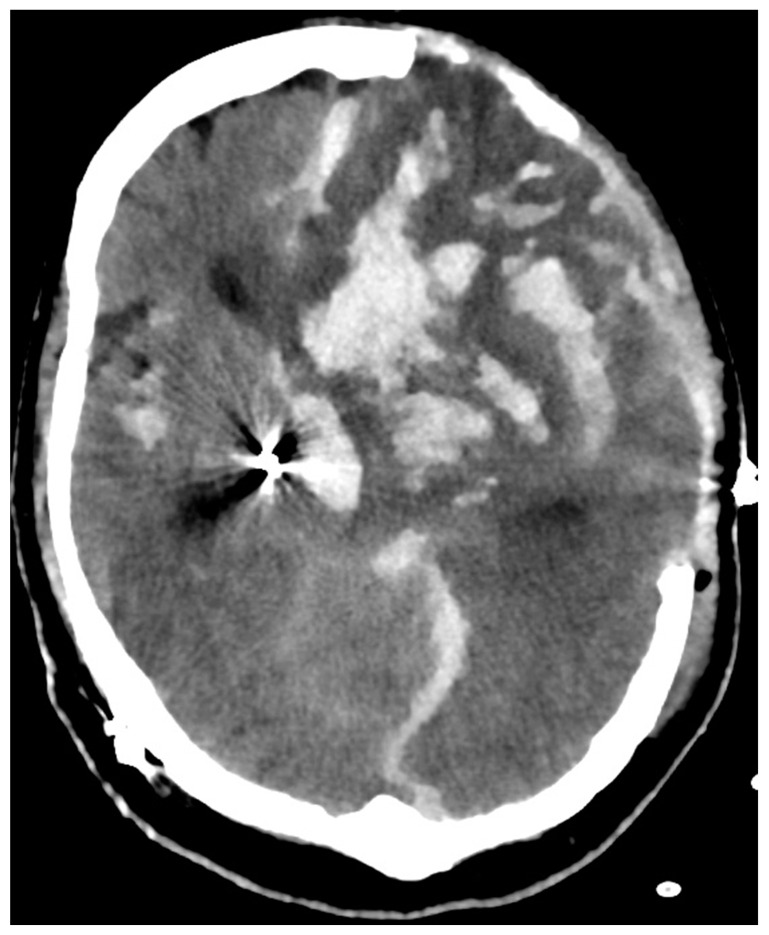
Postoperative head CT scan after clipping of the supra-ophthalmic aneurysm, left-sided DBS lead removal and decompressive hemicraniectomy. Eventually, therapy was switched to best supportive care due to massive parenchyma destruction.
